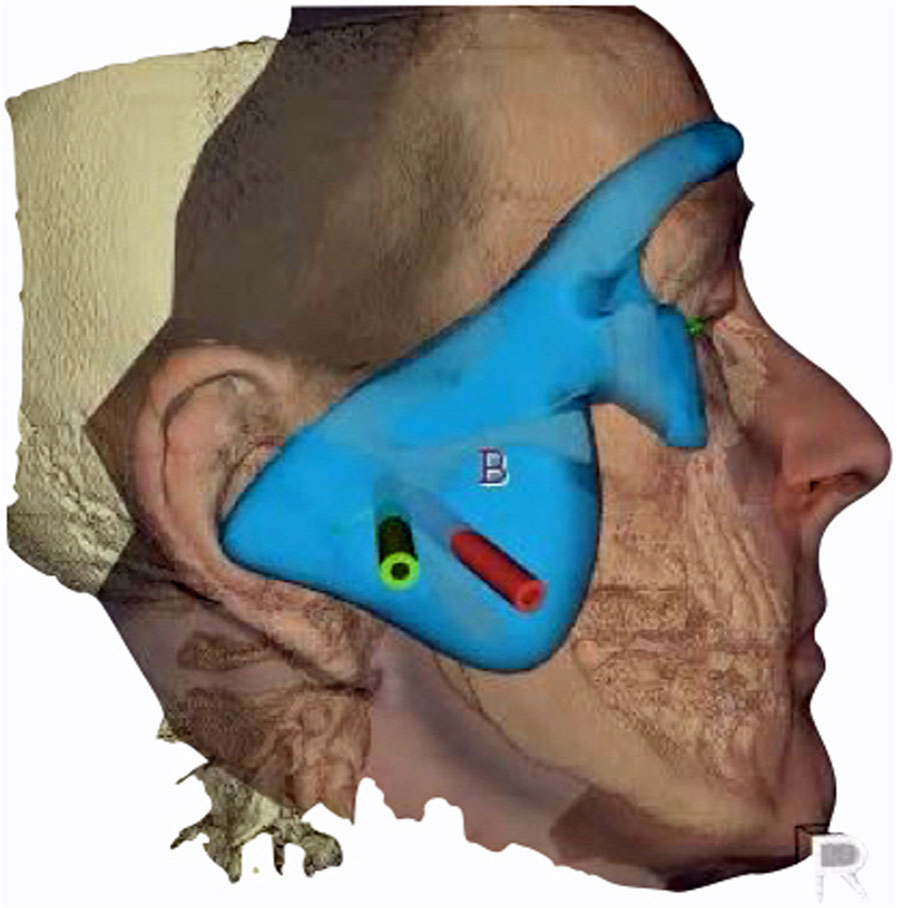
Surgical template prior to separation in 2 parts. The extensions to guarantee fit and the working channels are visible. Overlay of CBCT and optical scan
